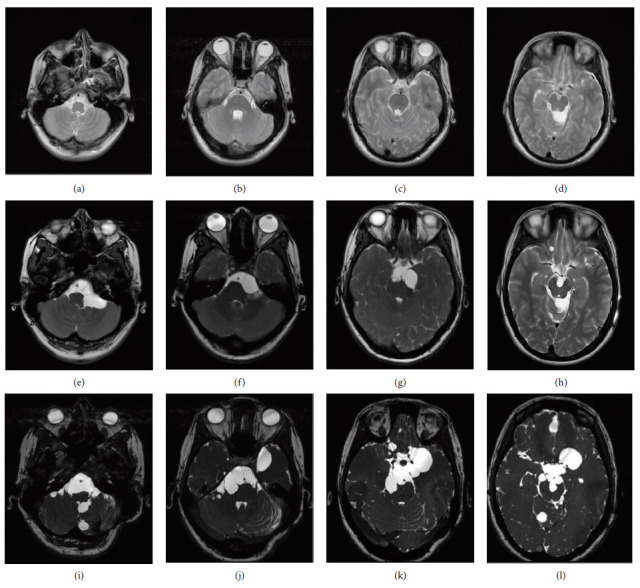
2010 T2-weighted (a)–(d), 2014 and 2020 FIESTA sequence ((e)–(h) and (i)–(l), respectively) magnetic resonance imaging (MRI). Slices at the level of the medulla oblongata (a), (e), (i), mid-pons (b), (f), (j), upper pons (c), (g), (k), and midbrain (d), (h), (l) showing the progressive increase in size of cysts.
